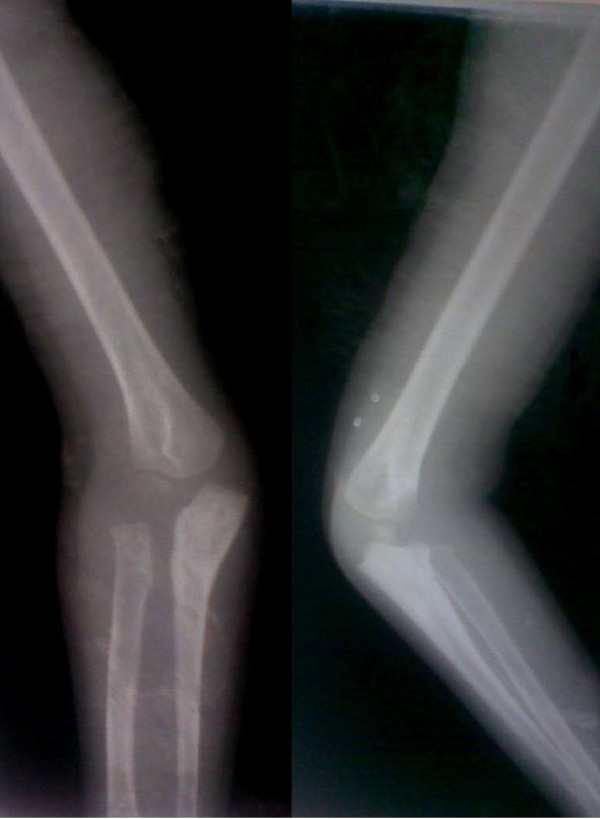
Preoperative radiograph of patient 1 showing Monteggia fracture dislocation (Patient 1).
